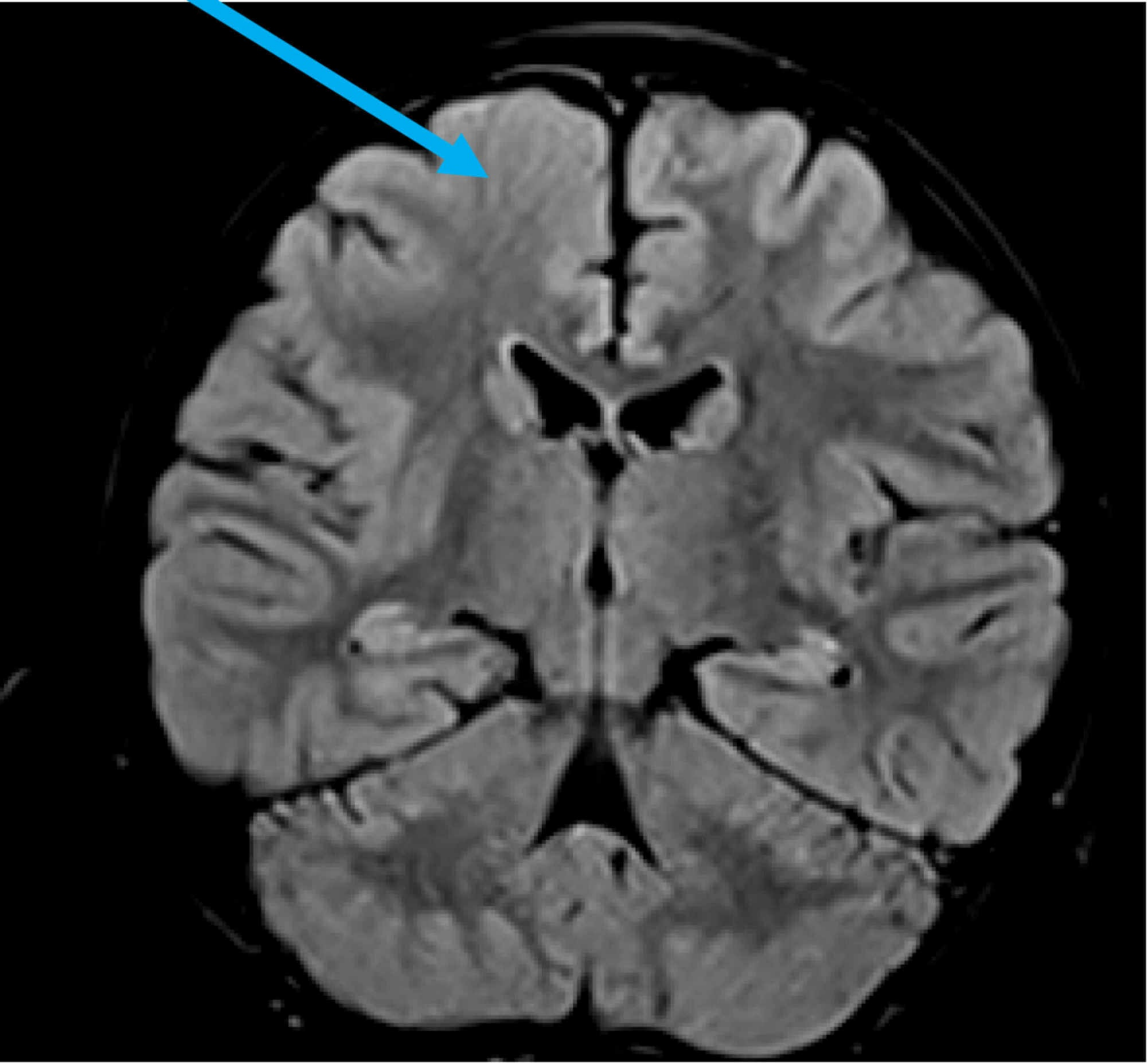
MRI findings of a six-year-old patient showed a focal cortical dysplasia on the right frontal pole (axial plane) MRI, magnetic resonance imaging
